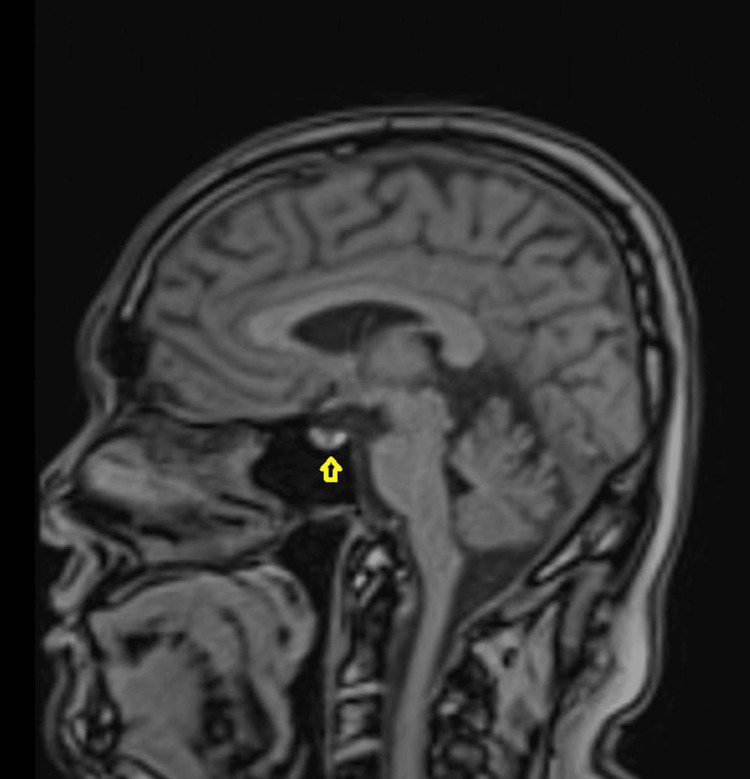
Sagittal T1-weighted MRI of the brain. The yellow arrow depicts the pituitary adenoma.
